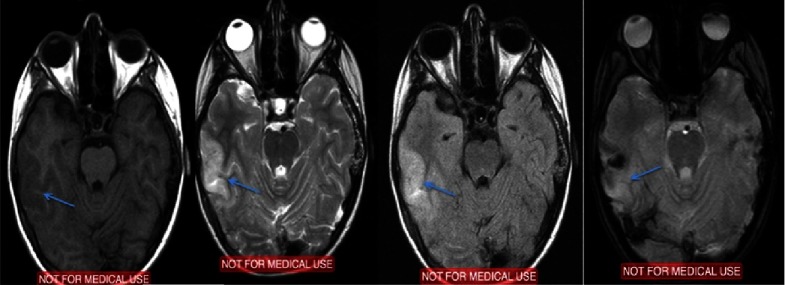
Initial MRI scan within one day after the event. Arrows depict the pathologic changes at T1W, T2W, FLAIR and T2 GRE sequences.
